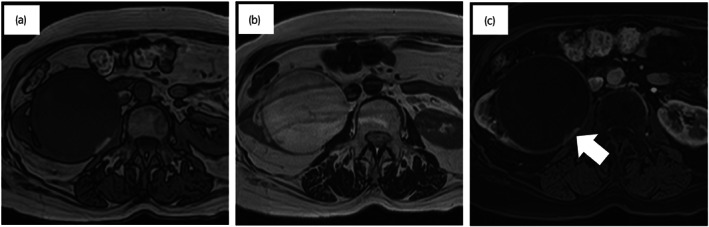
Abdominal MRI. (a) T1WI, (b) T2WI, and (c) dynamic contrast‐enhanced MRI. The arrow indicates an enhanced small nodule within the capsule of the tumor.
